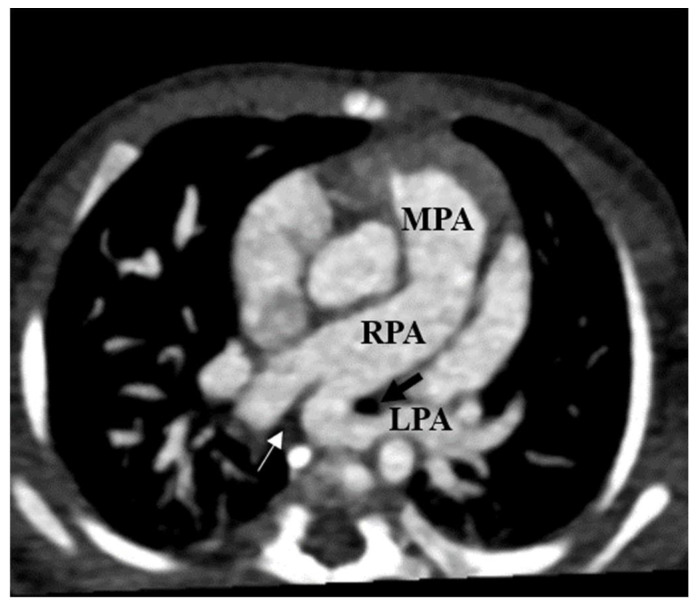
Left pulmonary artery sling (LPAS) in a 3-month-old girl with a cardiac murmur. Axial thin chest CTA shows an anomalous left pulmonary artery (LPA) arising from the posterior part of the proximal right pulmonary artery (RPA) and coursing between the left main bronchus (black arrow) and esophagus (thin white arrow) to the left pulmonary hilum.
